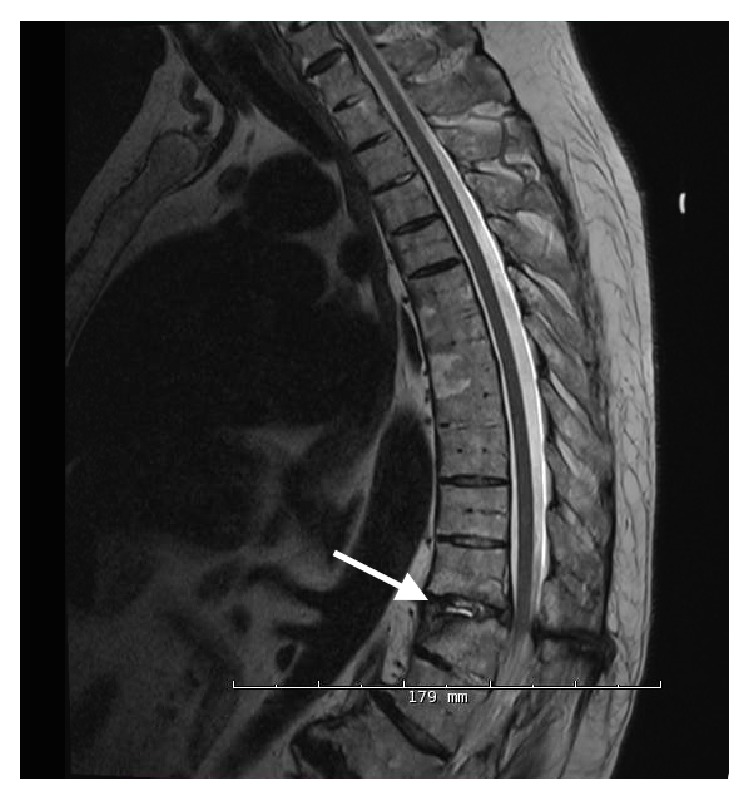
T2 thoracic MRI showing focal signal abnormality at the T11-T12 level (white arrow) with associated edema and loss of disc height, in addition to moderate spinal canal stenosis.
